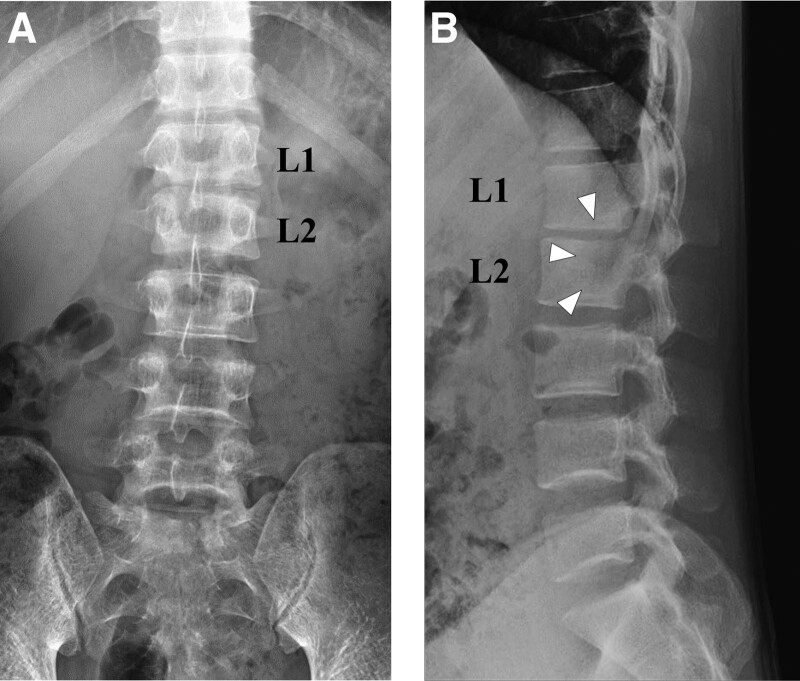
(A) Preoperative anterior–posterior view of plain radiograph showing a mild reduction in the L1–L2 intervertebral disc height. (B) Preoperative lateral view of plain radiograph showing mild osteolytic findings on the posterior side of the L2 vertebra (arrowheads).
